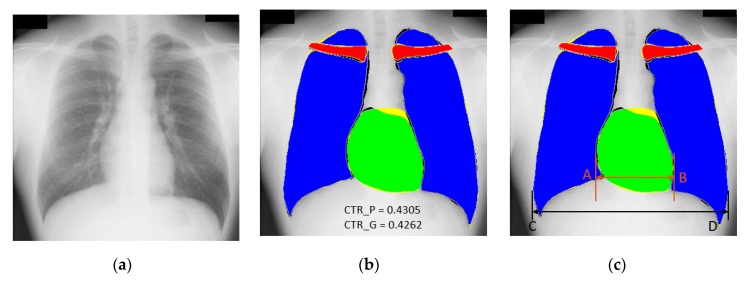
Sample image of chest anatomy segmentation for pixel count: (a) original image, (b) predicted mask by X-RayNet (*FP* (shown in black for each class), *FN* (shown in yellow for each class), and *TP* (shown in blue, green, and red for the lung, heart, and clavicle bone classes, respectively)), and (c) procedure for calculating CTR, CTR_P, and CTR_G represent the CTR predicted by the proposed method and that predicted by the ground-truth mask.
